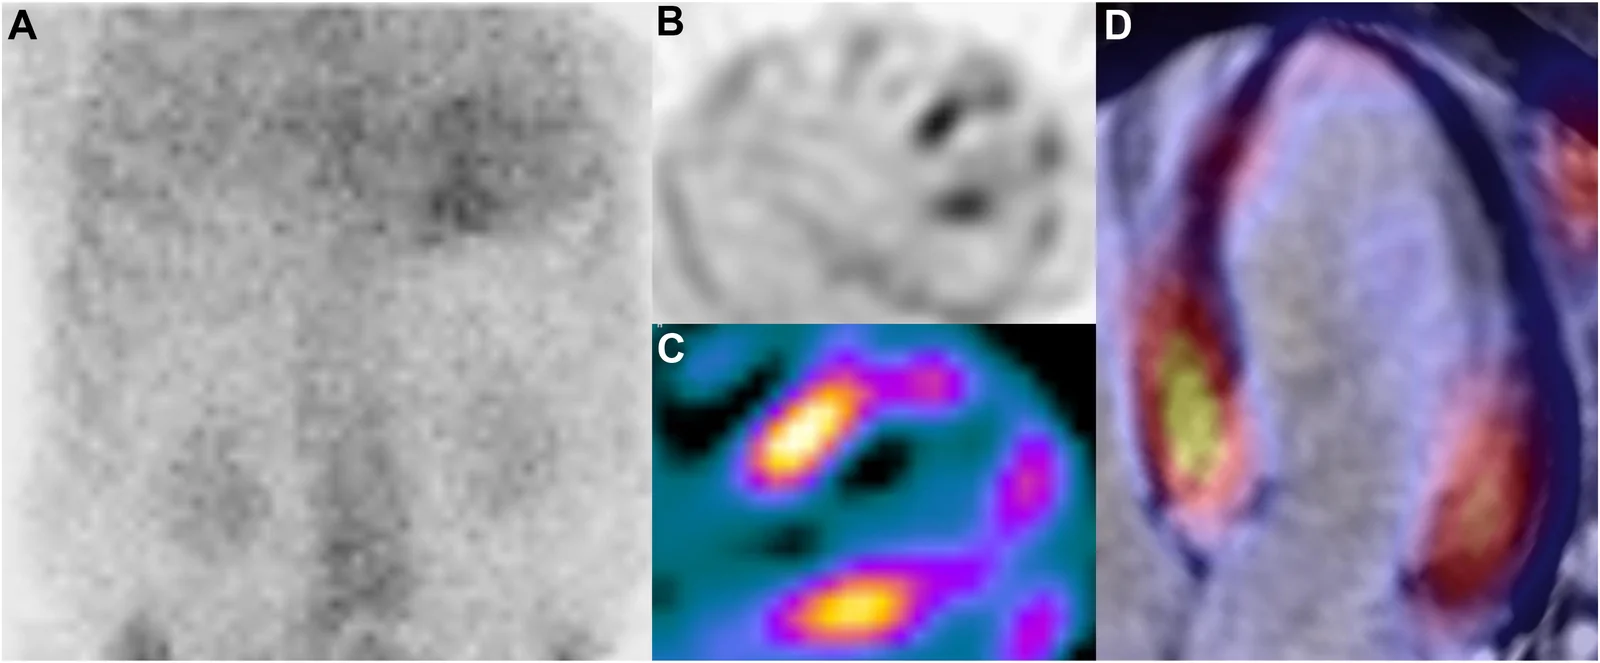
Technetium-99m Pyrophosphate Imaging in Cardiac Amyloidosis Cardiac amyloid radionuclide imaging performed at 1 hour with technetium-99m pyrophosphate shows a subtle abnormality on planar imaging (A) with activity adjacent to the sternum. On single-photon emission computed tomography images (B and C), nondiffuse (grade 2) myocardial uptake is clearly visualized in the basal left ventricle with more muted (grade 1) uptake in the mid-septum, closely matching the distribution of late enhancement on fused Cardiac amyloid radionuclide imaging/cardiac magnetic resonance late gadolinium enhancement images (D).
